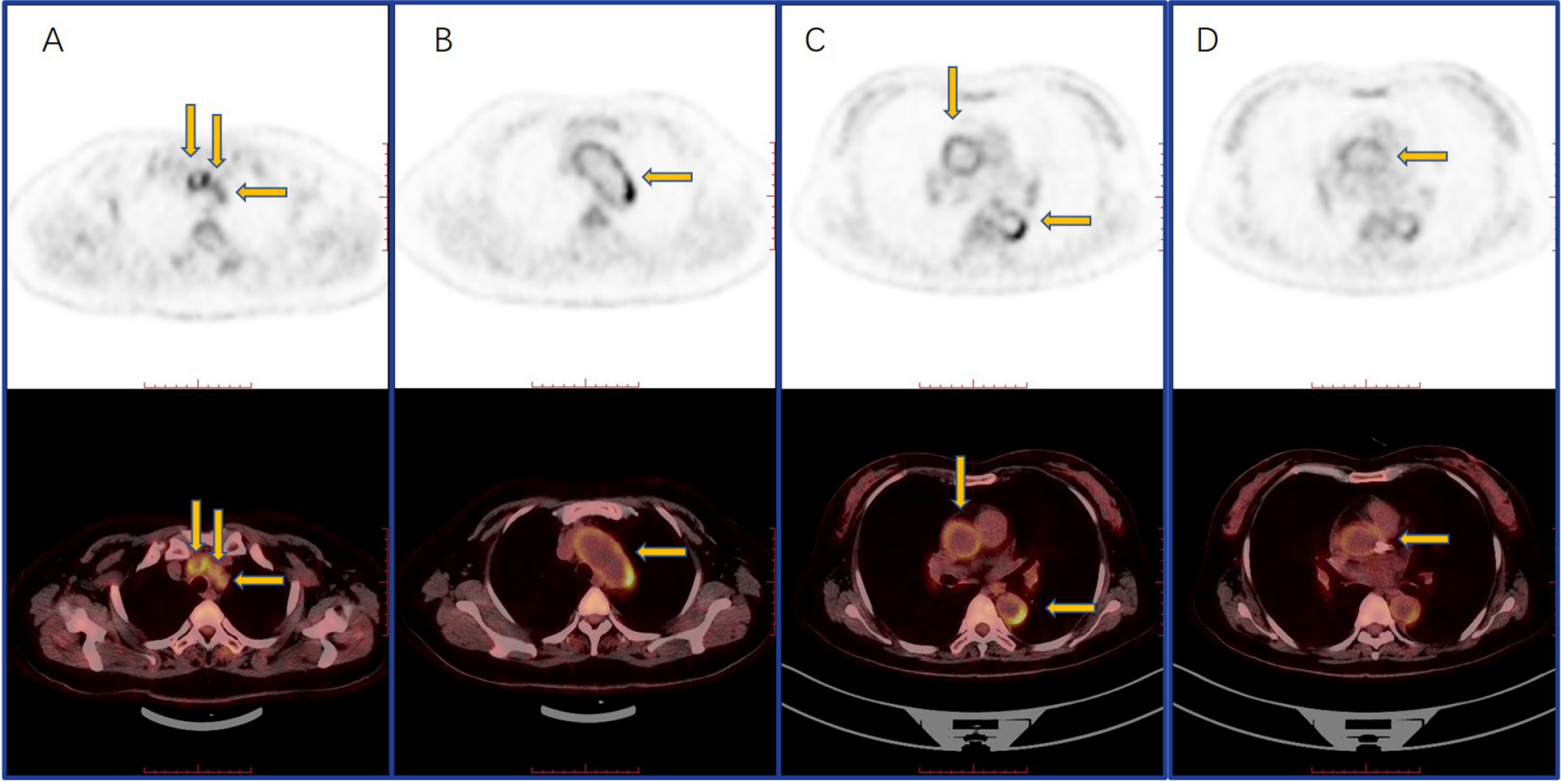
PET-CT showed an increased circular and linear 18F-FDG uptake in the subclavian and common carotid arteries (A), aortic arch (B), thoracic aorta (C), and coronary (D).
